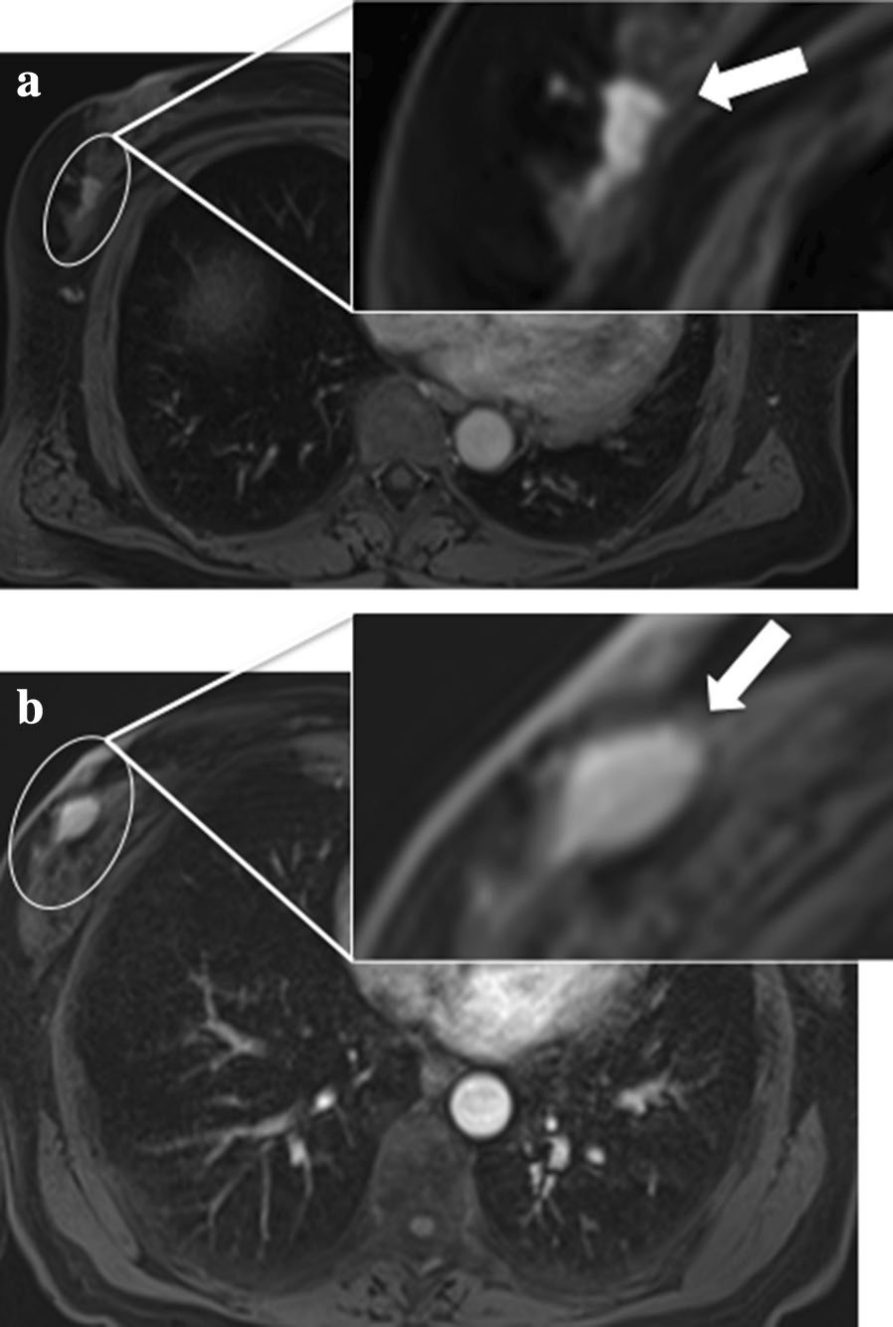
Examples of breast incidental findings by BI-RADS category. a A 67-year-woman (Patient 2 in Table 3) underwent abdominal MRI for follow-up of a cystic lesion of the head of the pancreas. Three-dimensional dynamic axial volumetric interpolated breath-hold examination image after intravenous power injection of 0.05 mmol/kg of Gadobenate dimeglumine (Multihance) at 60 s. More than 50 % of the breast tissue was visible at abdominal MRI. A suspicious mass (*white circle*) was found in the outer quadrants of the right breast (BI-RADS RM 4) The US-guided core needle biopsy confirmed a B5b lesion (Invasive Ductal Carcinoma). In the *upper right corner* is highlighted the breast findings (*white arrow*). b A 58-year-woman (Patient 3 in Table 3) underwent abdominal MRI for liver evaluation. Three-dimensional dynamic axial volumetric interpolated breath-hold examination image after intravenous power injection of 0.025 mmol/kg of gadoxetic acid (Primovist) at 60 s. More than 50 % of the breast tissue was visible at abdominal MRI. A suspicious mass (*white circle*) was found in the outer quadrants of the right breast (BI-RADS RM 4). This patient had a previously known breast cancer in the outer quadrants of the right breast, corresponding to the breast finding detected on MR images. In the *upper right corner* is highlighted the breast findings (*white arrow*)
